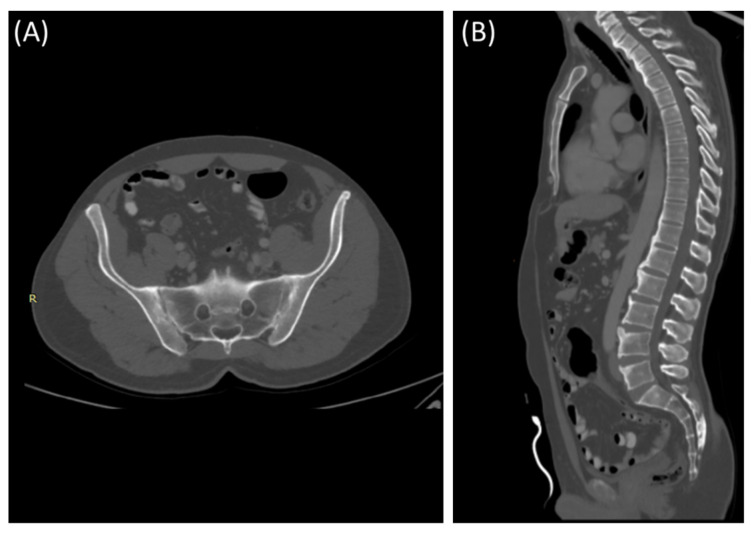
Axial bone window CT scan (A) showing Chronic sacroiliitis with ankyloses of the sacroiliac and a sagittal bone window CT scan (B) showing Syndesmophytes in the spine in a 55-year-old patient with Ankylosing spondylitis.
